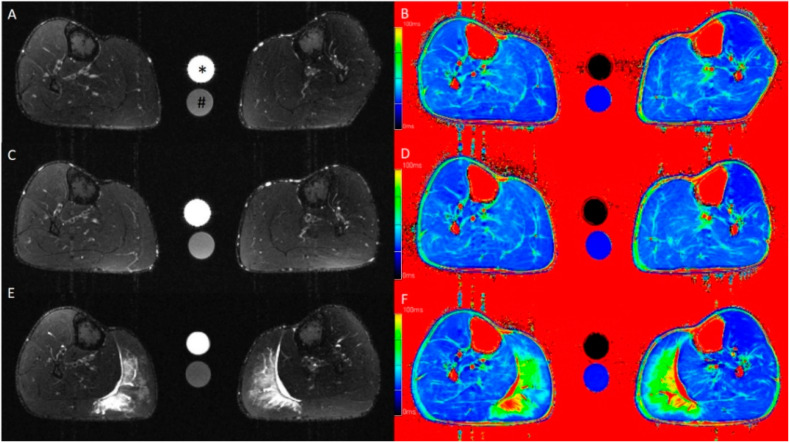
Axial T2-weighted turbo inversion recovery magnitude (A,C,E) and axial T2 mapping (B,D,F) images of the lower leg at baseline (A,B) and at 6 h (C,D) and 48 h (E,F) after standardized exercise of the calf muscles. Muscle edema is apparent in the medial gastrocnemius muscle in the post-exercise images after 48 h. This participant wore the compression garment on the left calf for 6 h after exercise. The calibration tubes contain 40 mmol/L NaCl (*) and 40 mmol/L NaCl with 5% agarose (#).
